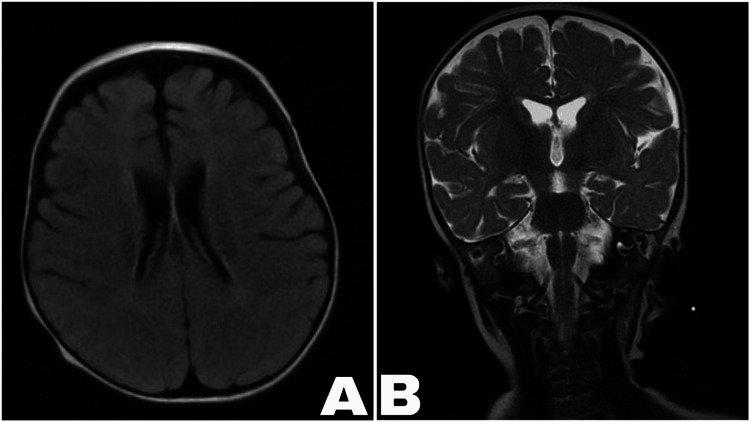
Brain MRI: (A) cross-sectional and (B) coronal images both revealed no abnormality MRI: magnetic resonance imaging
